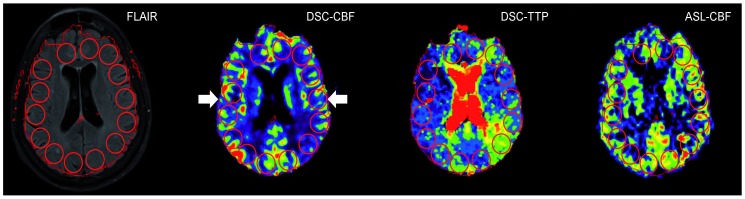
Quantitative analysis of DSC-CBF, DSC-TTP and ASL-relCBF. 20(ROIs) were placed on the FLAIR image and copied onto coregistered DSC-CBF, DSC-TTP and ASL images. For relative values, the ratio of each ipsilateral ROI to the arithmetic mean of all contralateral ROIs was calculated. In case of DSC-TTP the values were subtracted to obtain relative TTP-delay. DSC-perfusion maps were automatically masked by the used software. Therefore, the contour of DSC maps was used to define the cortical rim (contour, arrows).
